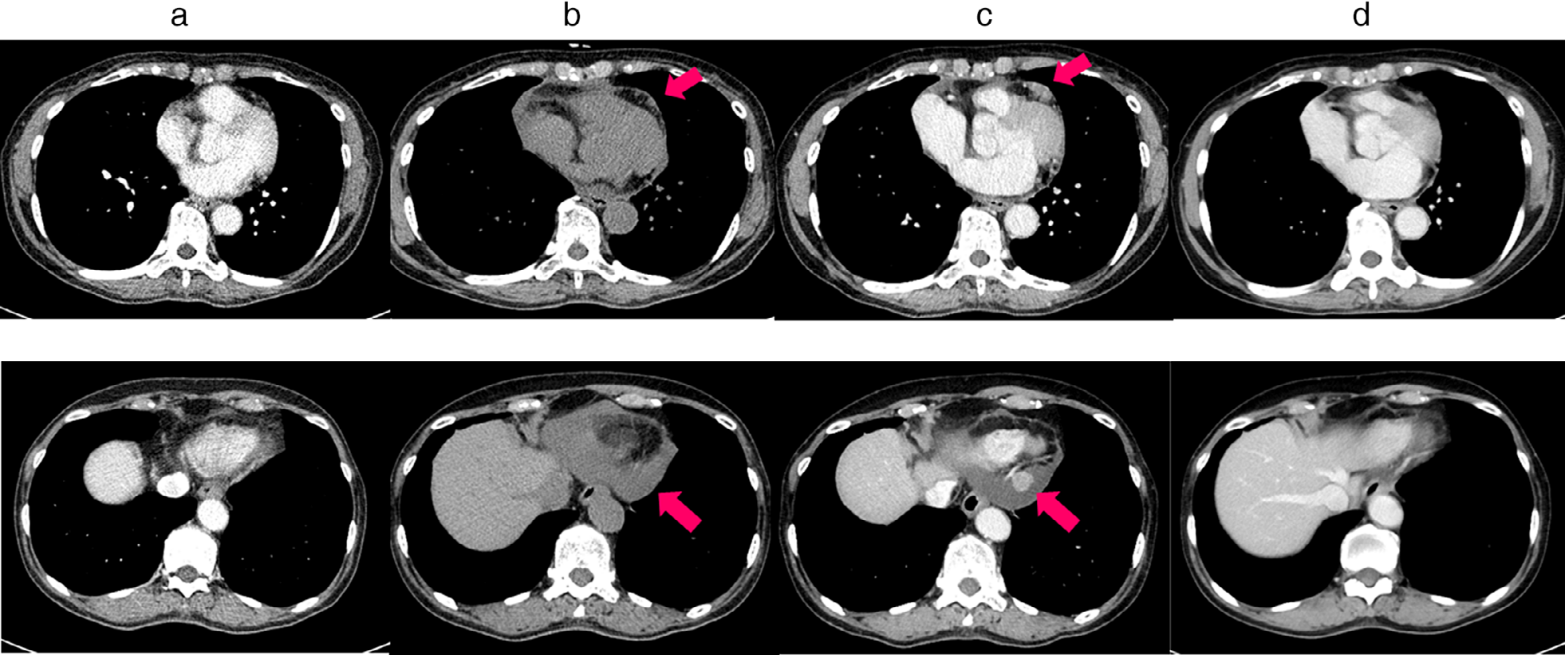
(a) The upper image shows computed tomography of the chest showing 7 cm below the carina. The lower image shows 1 cm above the pericardial end. There were no nodules in the pericardium before lung cancer surgery. (b) Several small nodules were observed in the pericardial space at six months after lung cancer surgery. (c) The nodules grew and pericardial effusion increased at eight months after lung cancer surgery. (d) All nodules had naturally disappeared at two months after surgical biopsy.
